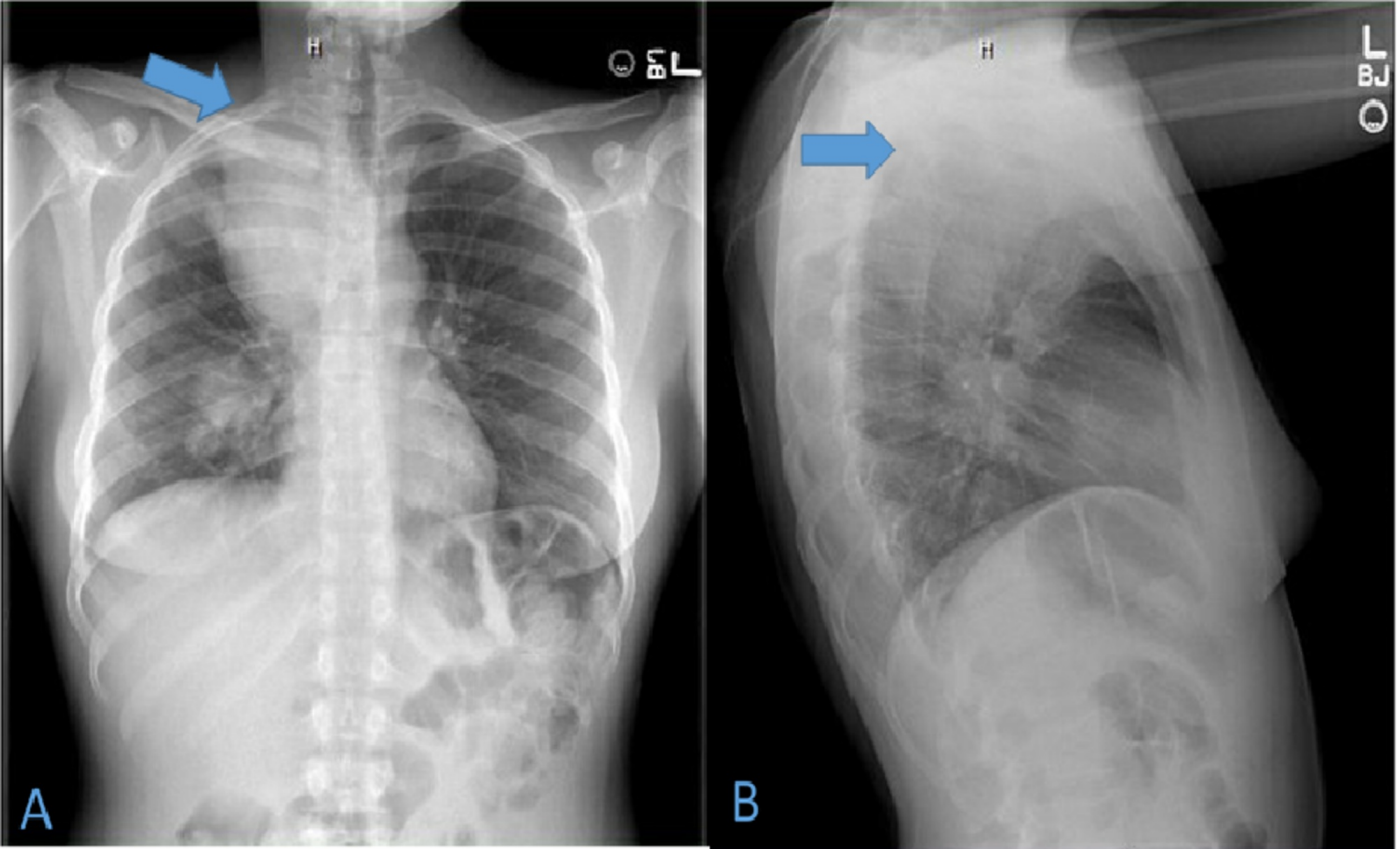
Postero-anterior and lateral view of chest X-ray 1A. Postero-anterior view of the chest showing the right upper lung mass, indicated by the blue arrow. 1B. Lateral view of the chest showing the right upper lung mass, indicated by the blue arrow.
